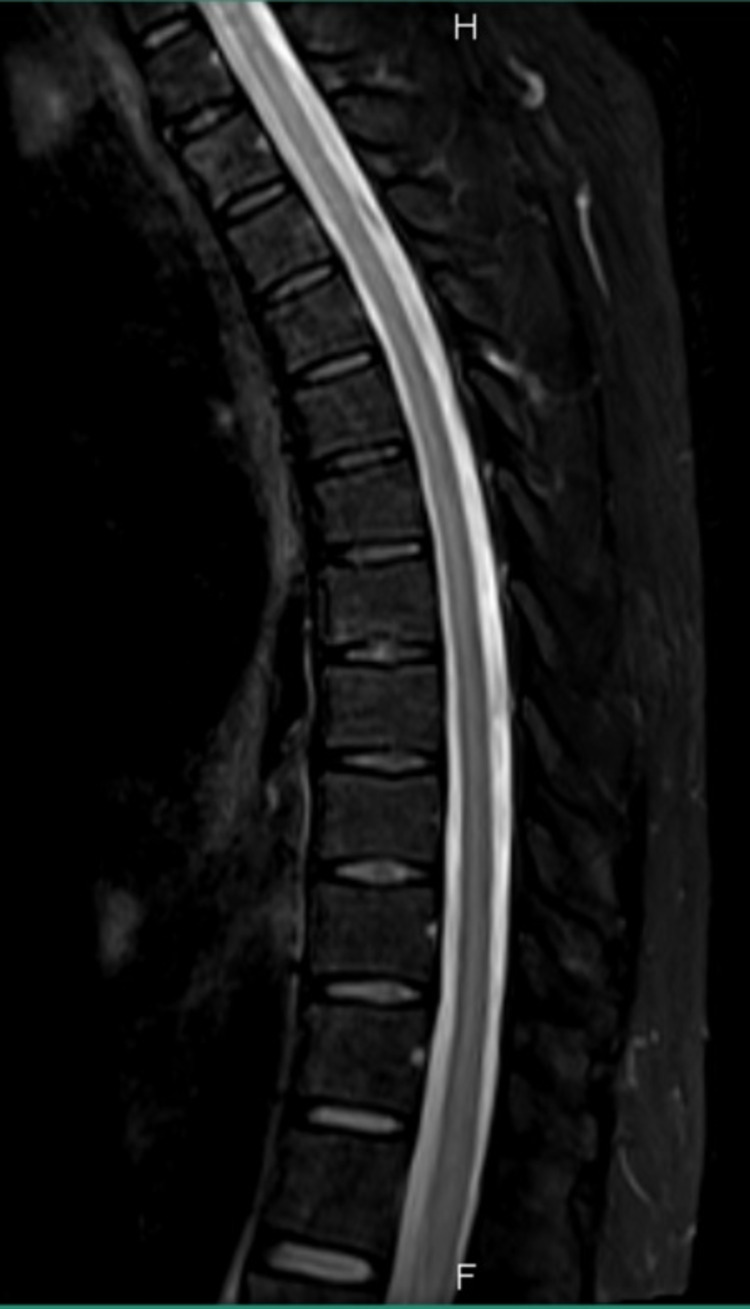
Sagittal short tau inversion recovery MRI of the dorsal spine posttreatment. The MRI demonstrates normal morphology and resolution of the signal intensity noted earlier. MRI: magnetic resonance imaging
